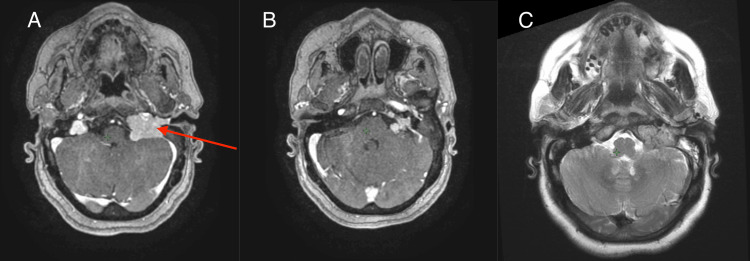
Cerebral MRI showing a left tympano-jugular tumor process (red arrow) measuring 39 x 29 mm responsible for osteolysis of the jugular foramen, with lobulated contours, containing flow voids on the T2 sequence with intense, homogenenous enhancement after contrast, evoking a paraganglioma A: T1 injected; B: T1 non-injected; C: T2
